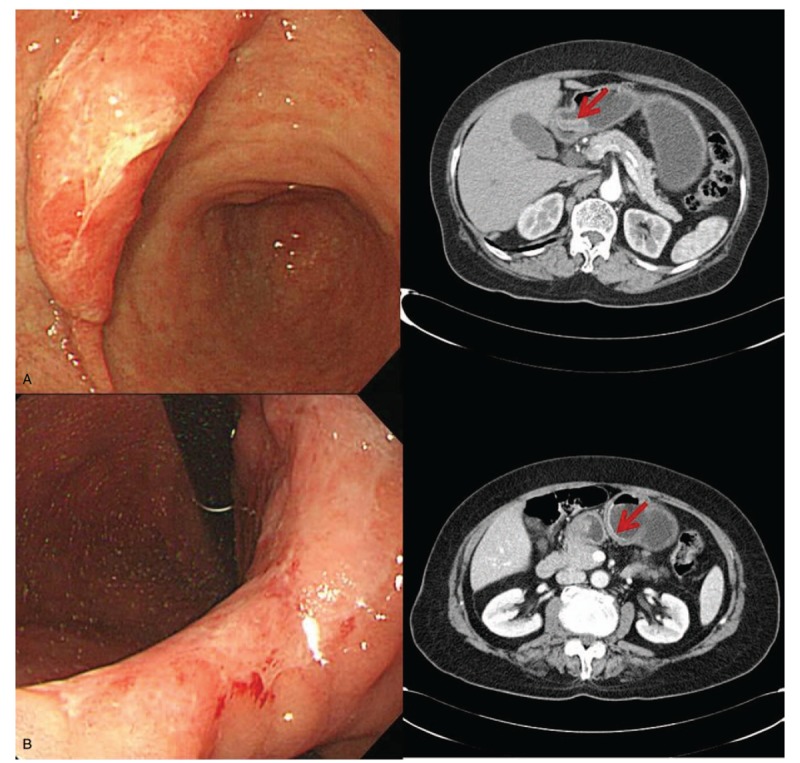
(A) 77-years-old-female patient. EGC with SM invasion was found as an elevated lesion in gastroscopy and wall thickening in CT scan. (B) 78-years-old-female patient. She had also EGC with SM invasion and shallow depressed lesion was found in gastroscopy. In CT scan, wall enhancement was noted.
